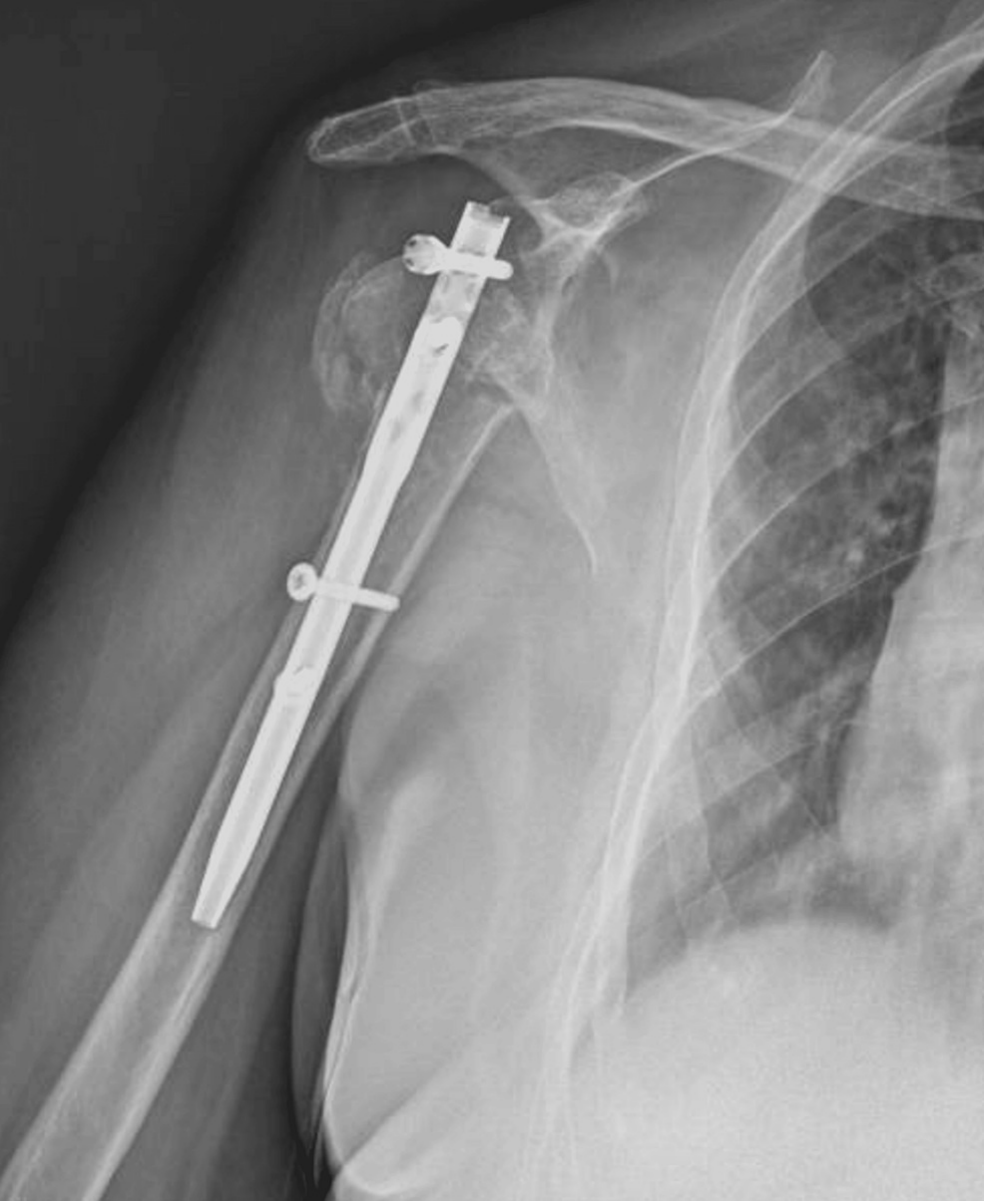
X-ray for evaluation of nail osteosynthesis failure (Case 2)
